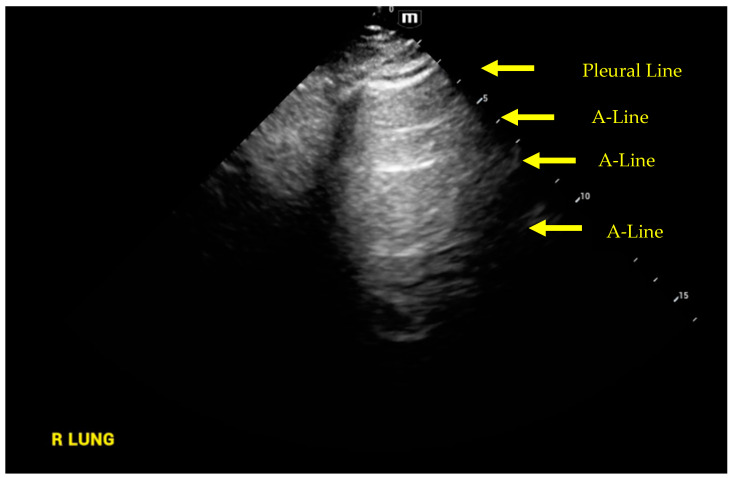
Normal lung ultrasound, A-line pattern.
